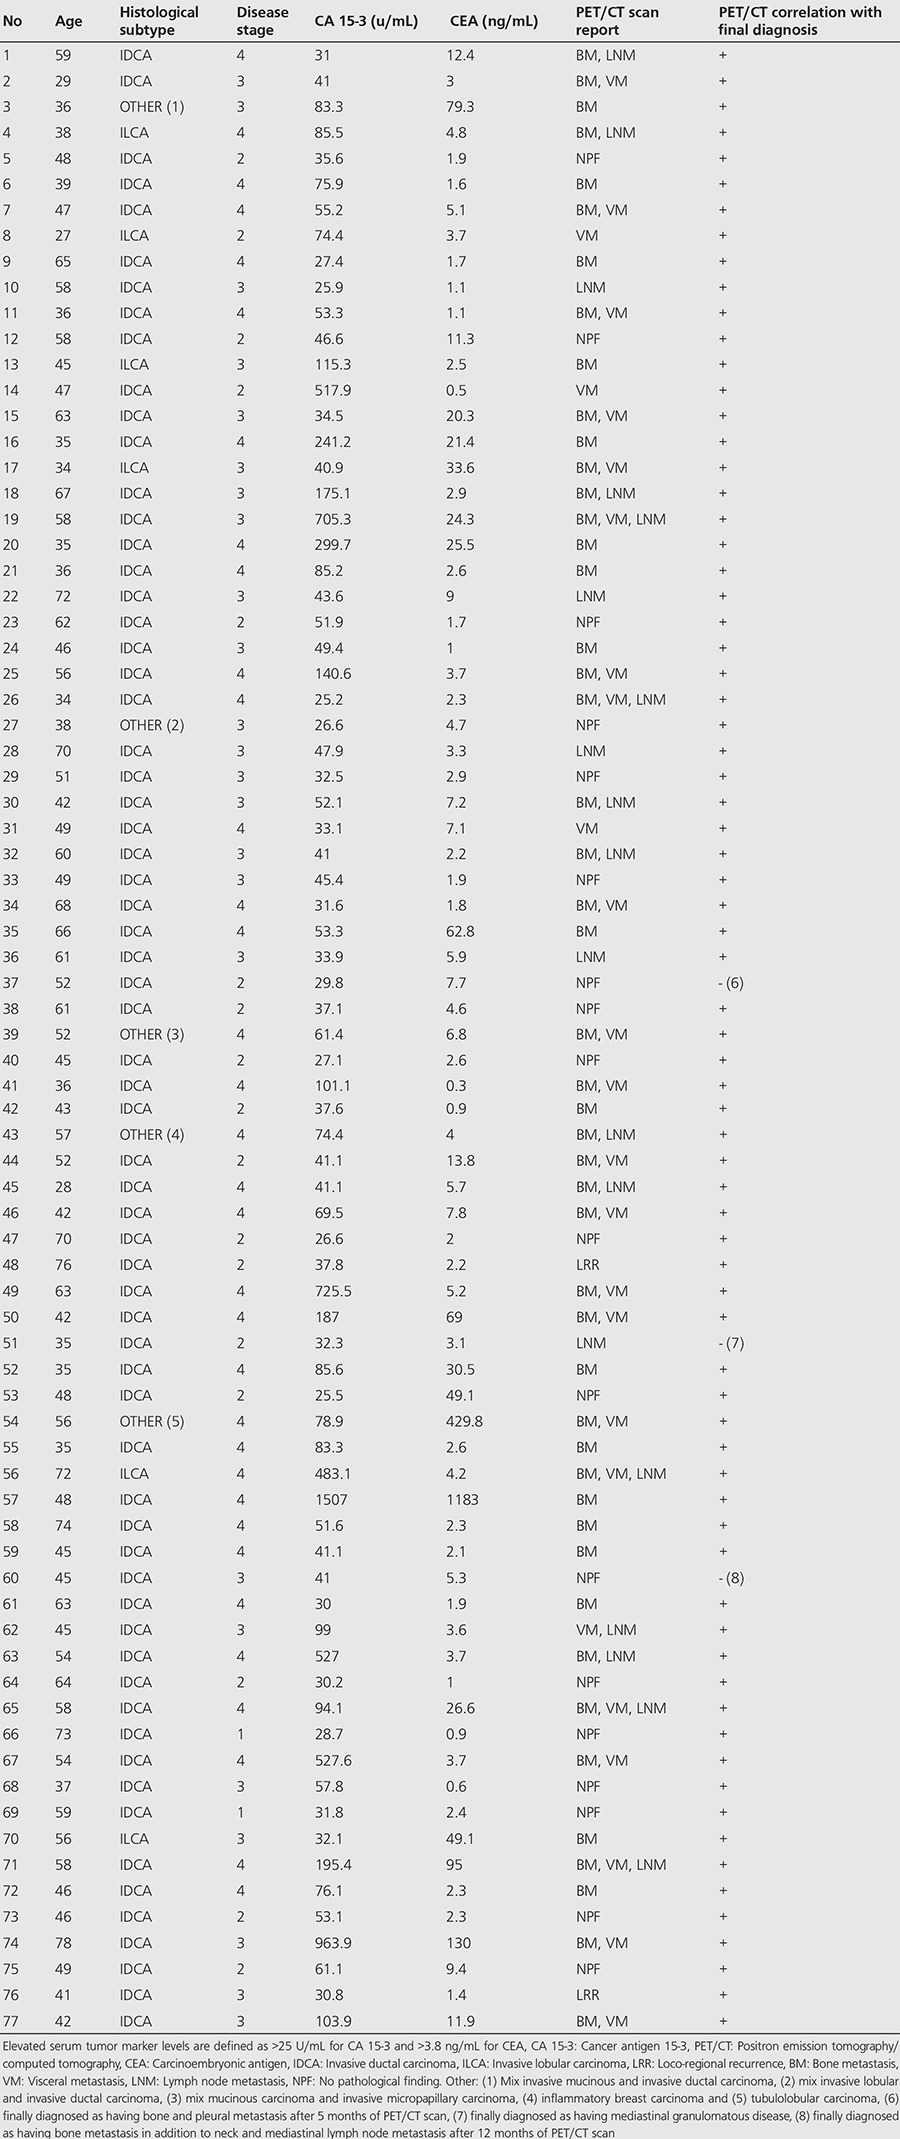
Patient characteristics and positron emission tomography/computed tomography scan results
